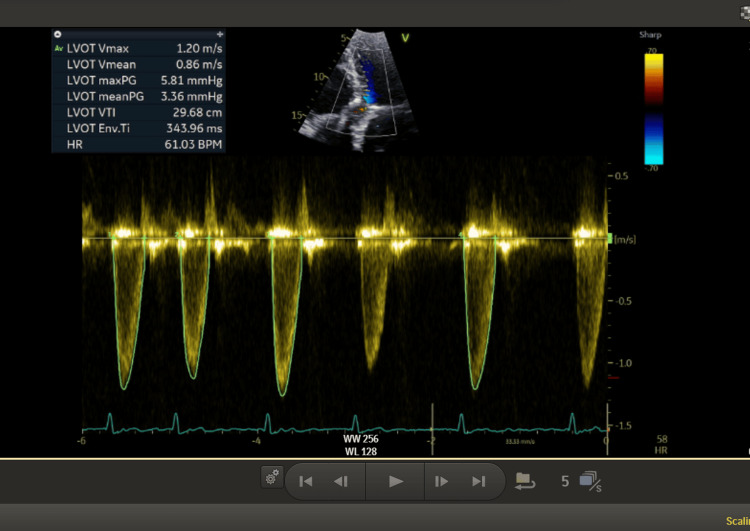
Continuous wave Doppler at the aortic area showing an aortic valve peak velocity of around 2.0 m/s
